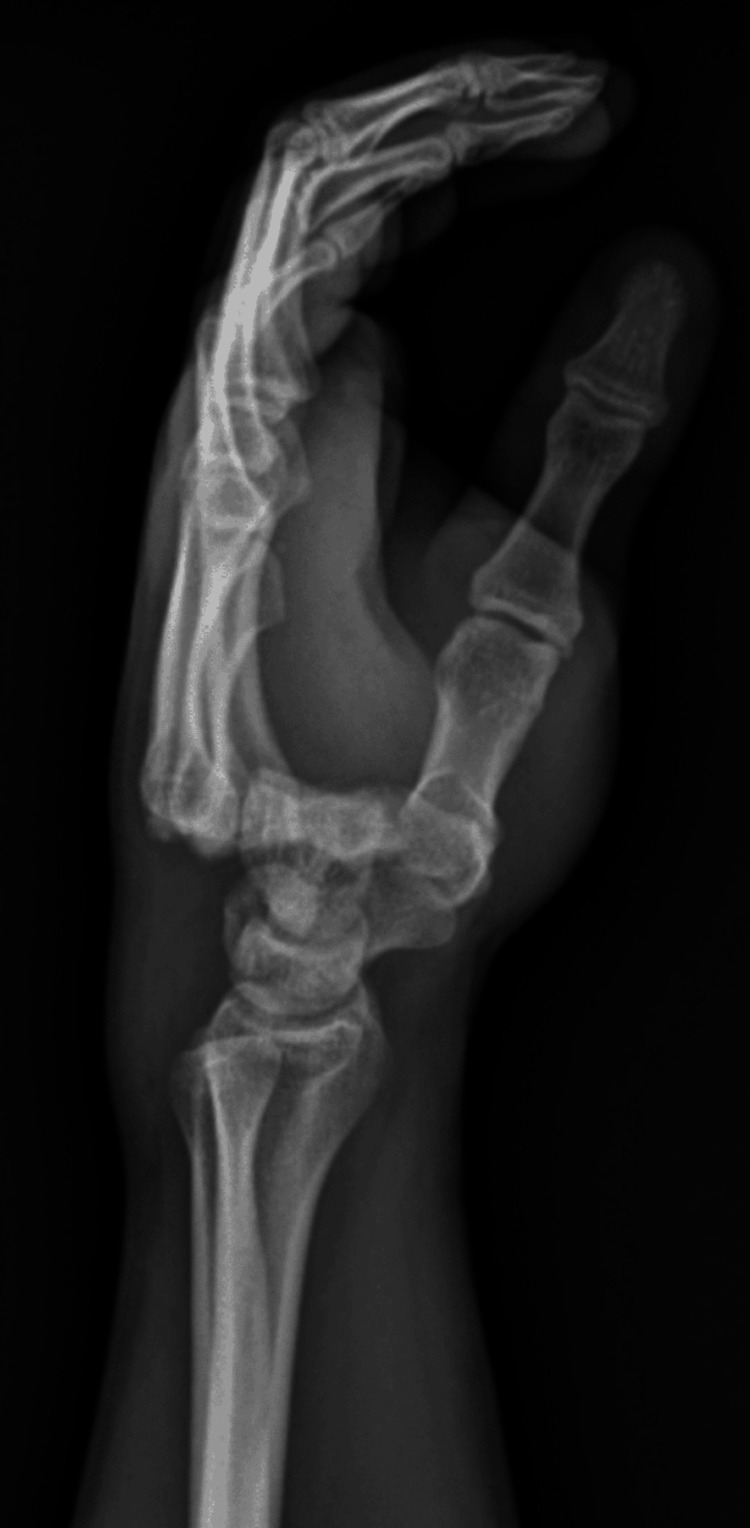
Lateral X-ray view of the carpometacarpal joint demonstrating dorsal dislocation
